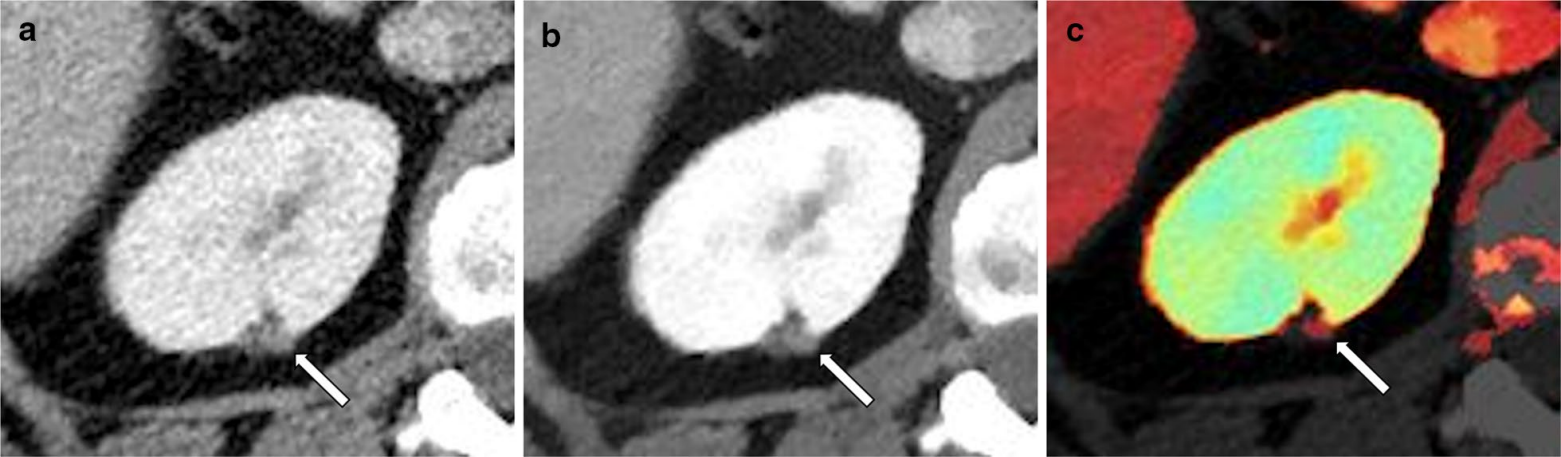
A 54-year-old woman underwent a routine abdominal CT before hernioplasty. Conventional images showed an incidental finding in the right kidney (arrows) with heterogeneous attenuation on the portovenous phase (a). The lesion’s heterogeneity and suspected enhancing component are accentuated using a 50 keV mono-energetic image (b). Iodine maps prove that the lesion’s high attenuation is true enhancement (c), showing iodine content of 4.8 mg/mL and a normalized iodine content of 1.1 mg/mL, suspicious for RCC
